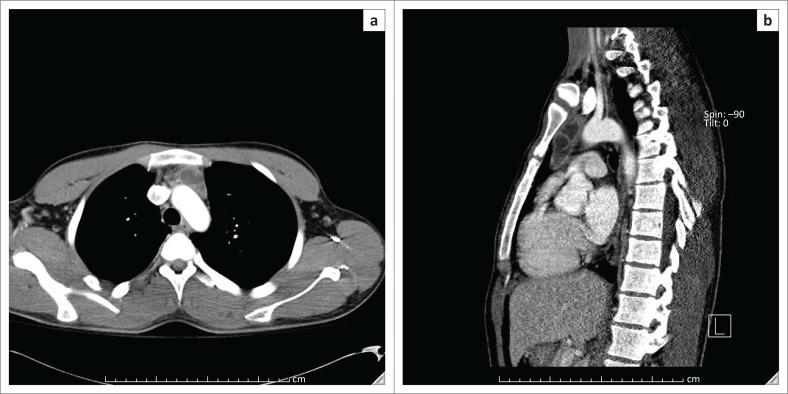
Axial (a) and sagittal (b) contrast enhanced computed tomography imaging of the chest demonstrates a well-defined cystic and solid mass containing punctate calcifications in the anterior mediastinum, without mass effect on the adjacent vascular structures.
